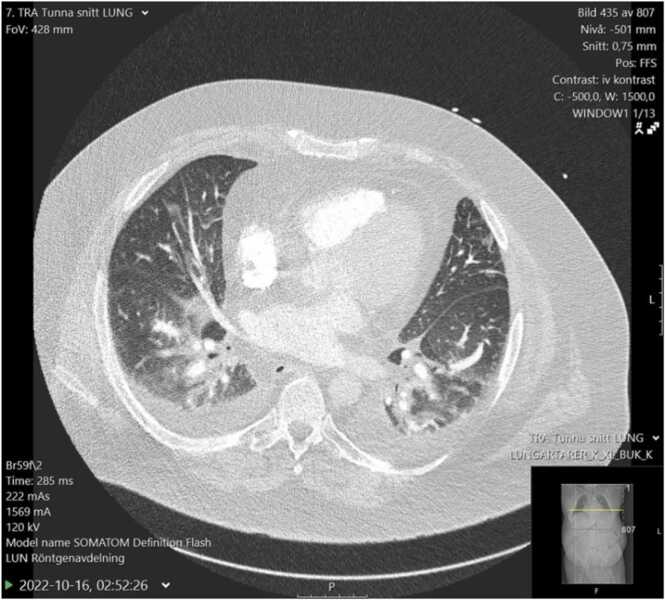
CT scan with signs of pulmonary congestion, bilateral pleural effusion and bilateral peribronchial parenchymal infiltrates.
